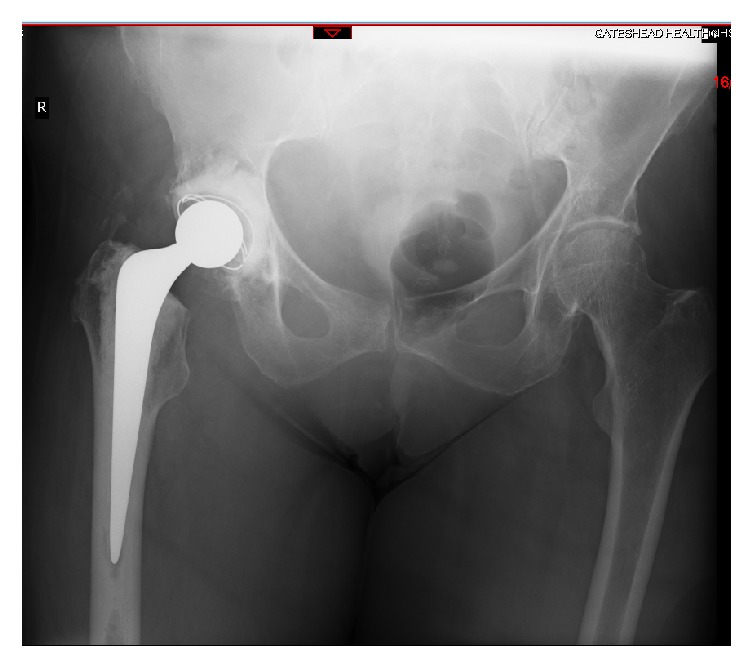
Plain X-ray of the right hip with normal soft tissues and satisfactory position of prosthesis.
